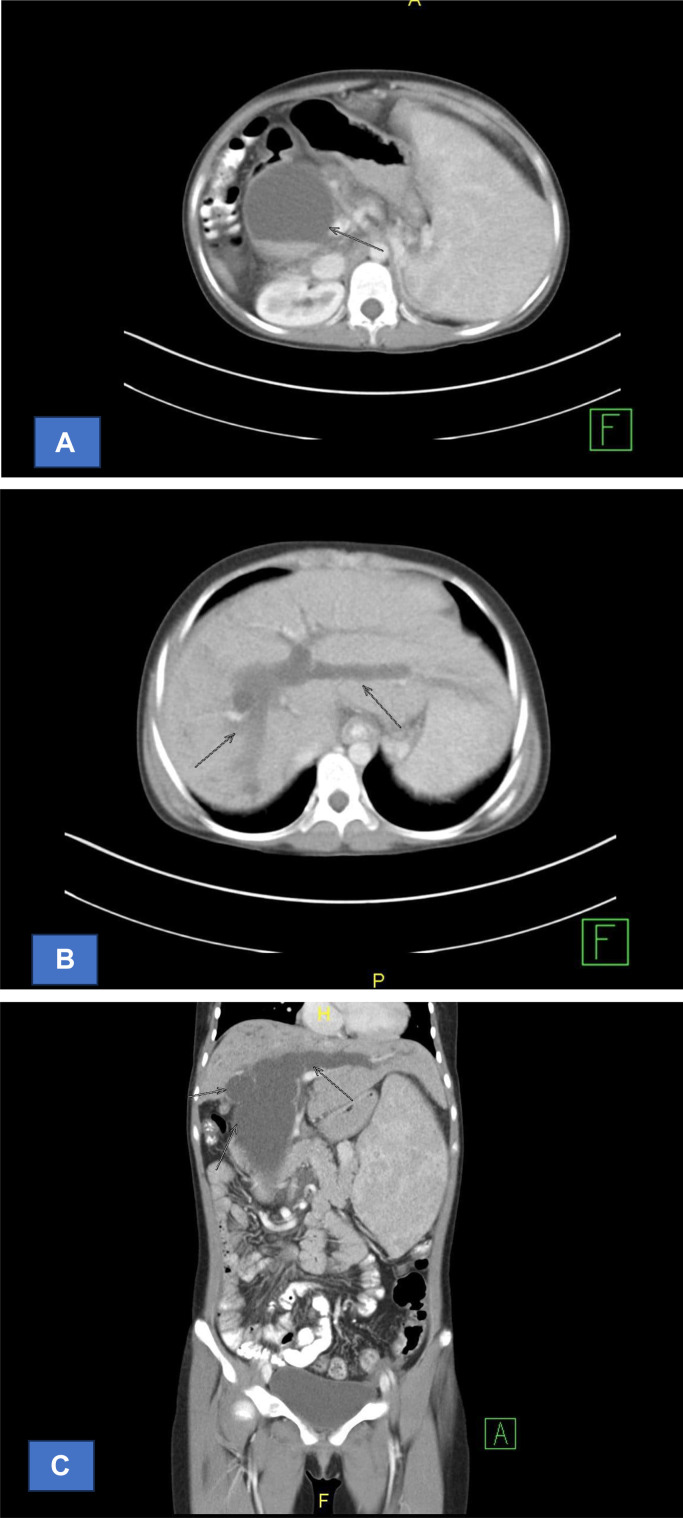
CT scan: sagital intra and extra hepatal duct, patients with Choledochal Cyst type IV: (A) The axial cut shows the dilation of the intra and extrahepatal ductus, (B) The axial cut shows the dilation of the intahepatal ductus, (C) The coronal cut shows the dilation of the intahepatal ductus, size of the lesion has not increased, the wall is not thickened, and the edges are regular. No masses or stones are visible. The extrahepatic bile duct is dilated, with a cystic lesion containing bile sludge, measuring approximately 6.25 × 7.14 × 12.40 cm, located in the proximal to distal common bile duct.
